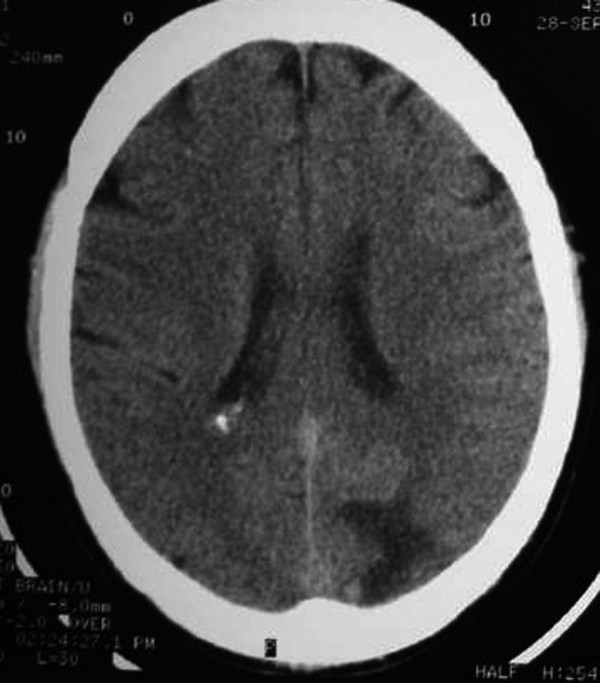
Brain CT without contrast: left occipital cortico-subcortical hypodense area.
